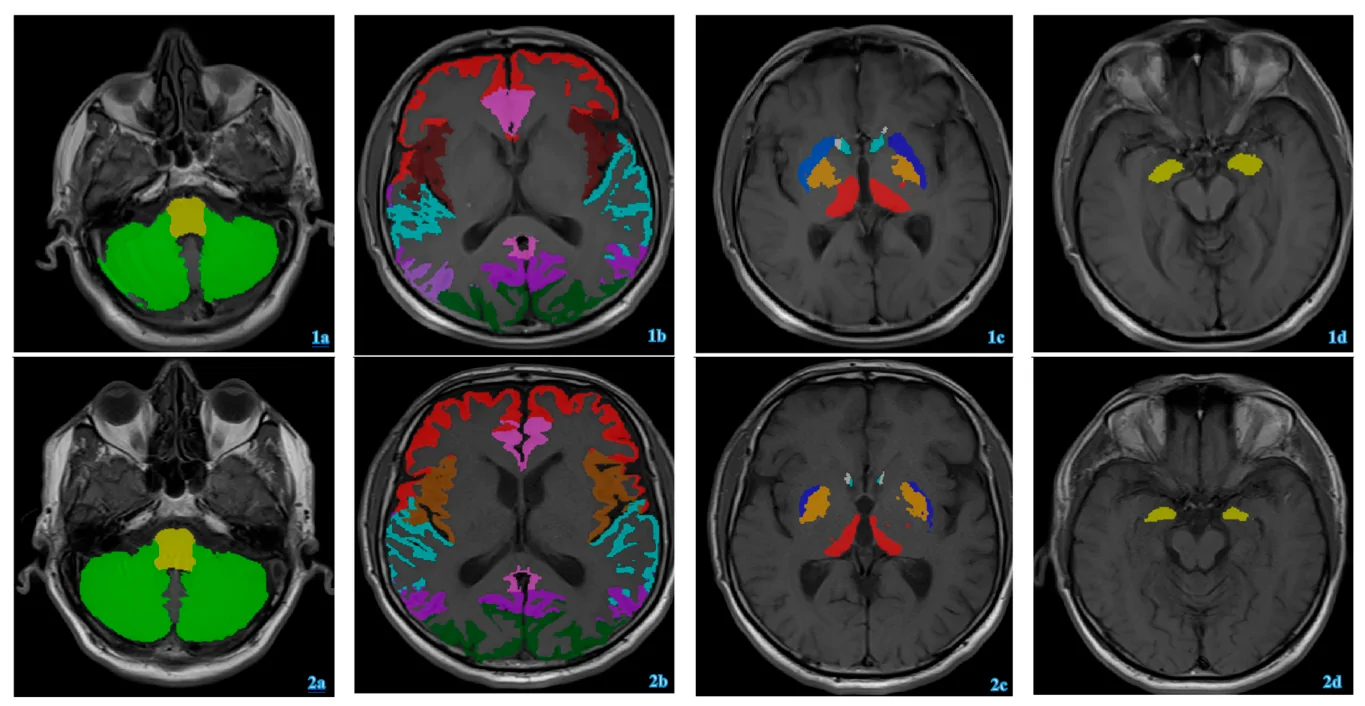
A patient’s MRI-1:a/MRI-2:b: (1a,2a) cerebellum light green and brainstem yellow; (1b,2b) frontal red, temporal turquoise, parietal purple, occipital dark green, limbic pink, and insular brown; (1c,2c) putamen blue, caudate white, pallidum orange, thalamus red, and accumbens turquoise; (1d,2d) amygdala yellow.
